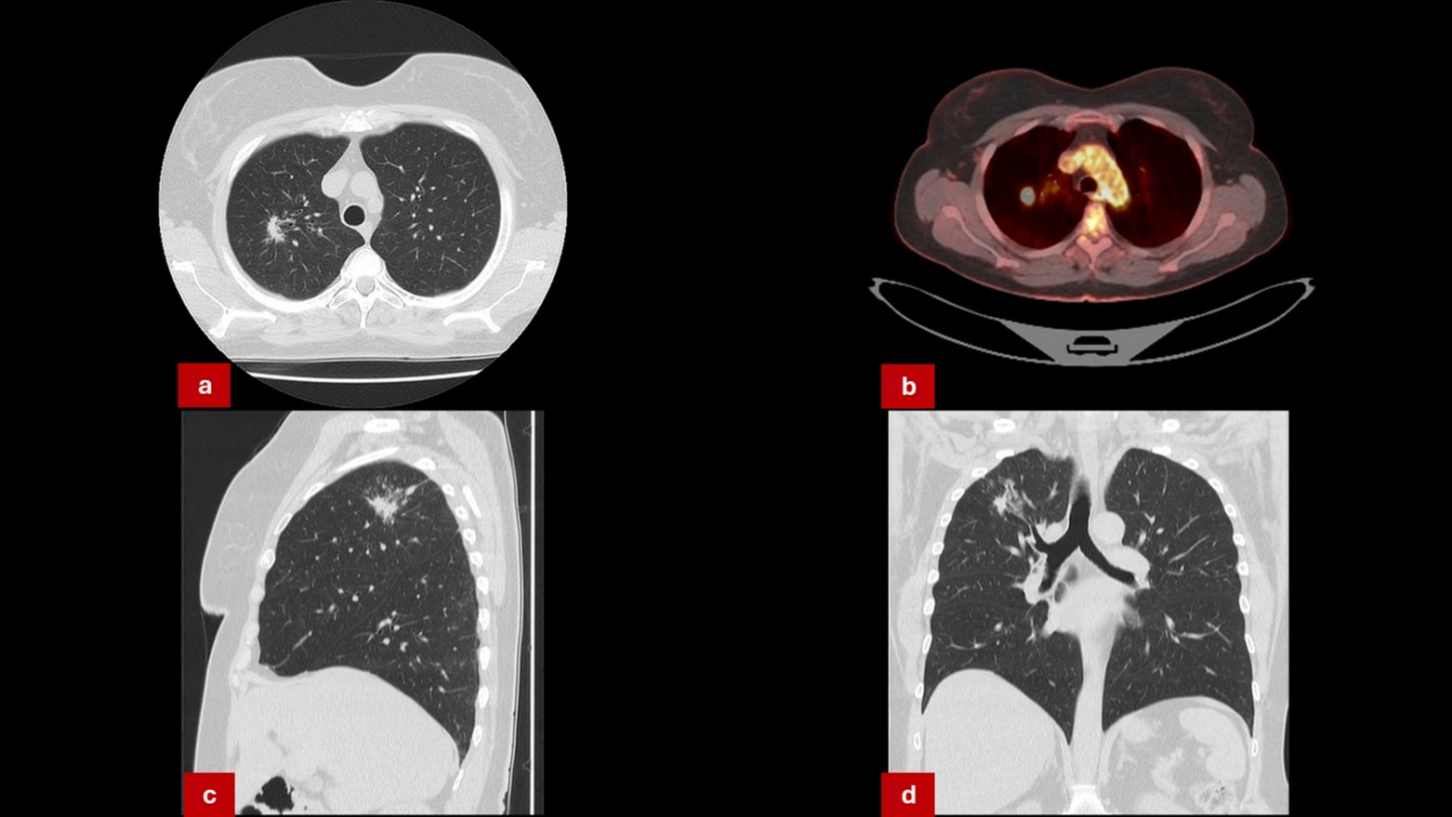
(a, c, d) CT‐scan revealing suspicious lesion in right upper lobe. (b) PET‐CT scan showing avid uptake in the right upper lobe lesion.
